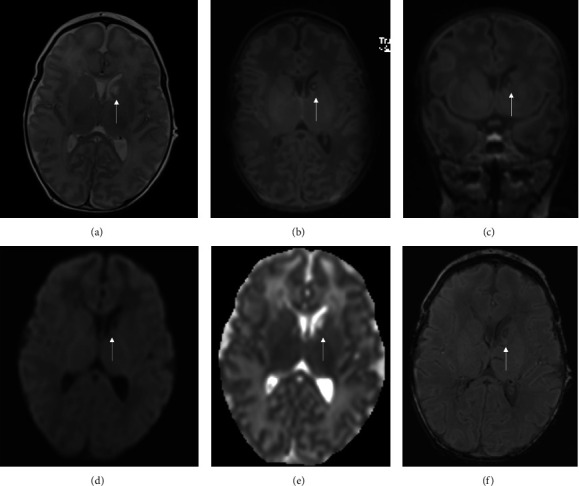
Magnetic resonance imaging (MRI) findings on the 15th day of life. MRI findings reveal a postischemic lesion in the left Caput nuclei caudati, consistent with striatal lacunar infarction with small central bleeding (white arrow). Axial T2 sequence shows white matter hyperintensity (a). Corresponding intensity on axial (b) and coronal (c) T1-weighted sequence. High-signal intensity at apparent diffusion coefficient (ADC) map (d) and low-signal intensity at diffusion-weighted imaging (e). Beginning of transformation at susceptibility-weighted imaging (SWI) (f).
